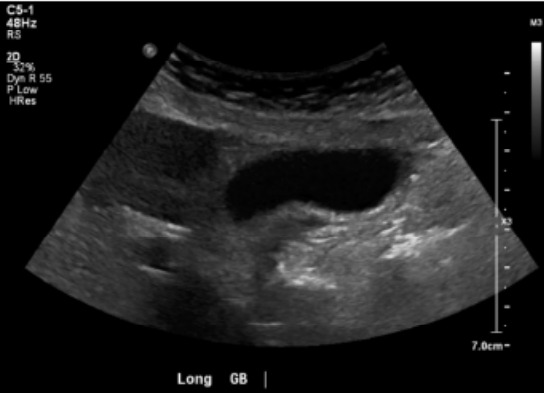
Right upper quadrant ultrasound of a 70-year-old woman with chest tightness and bradycardia.
